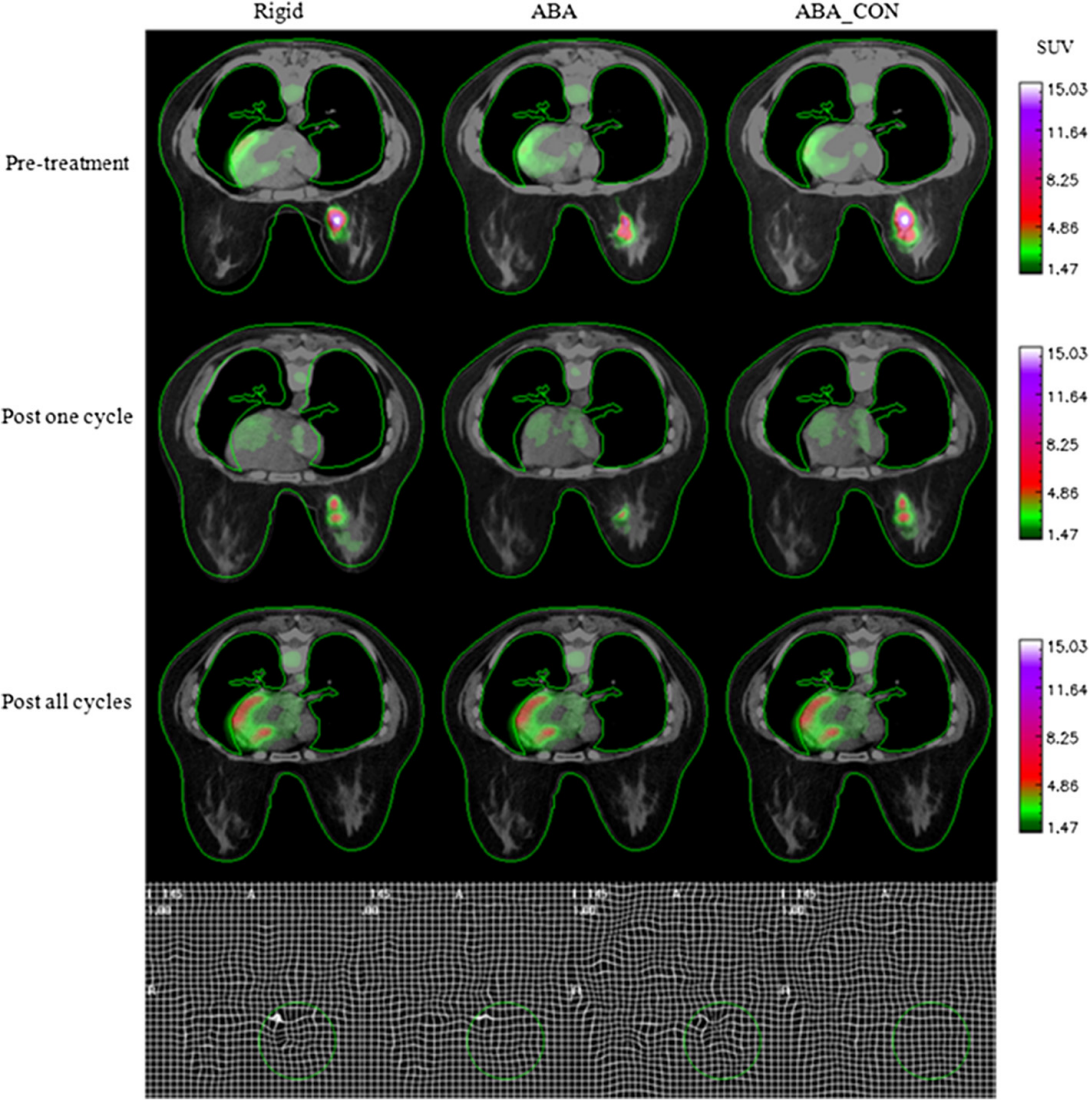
A representative patient displaying the results of the three registration algorithms. The first three rows correspond to the three time points, and the three columns show the results after rigid body registration, after unconstrained ABA registration (ABA), and with constrained ABA registration (ABA_CON), respectively. In the fourth row, the first panel displays the deformation field generated by the ABA when the images at *t*_1_ are registered to the images at *t*_3_, while the second panel shows the result using the ABA_CON; the third and fourth panels display similar data when the images at *t*_2_ are registered to the images at *t*_3_, respectively. The green circle shows the tumor location. The contour of the CT image at *t*_3_ is drawn and then copied to other images to facilitate the comparison.
